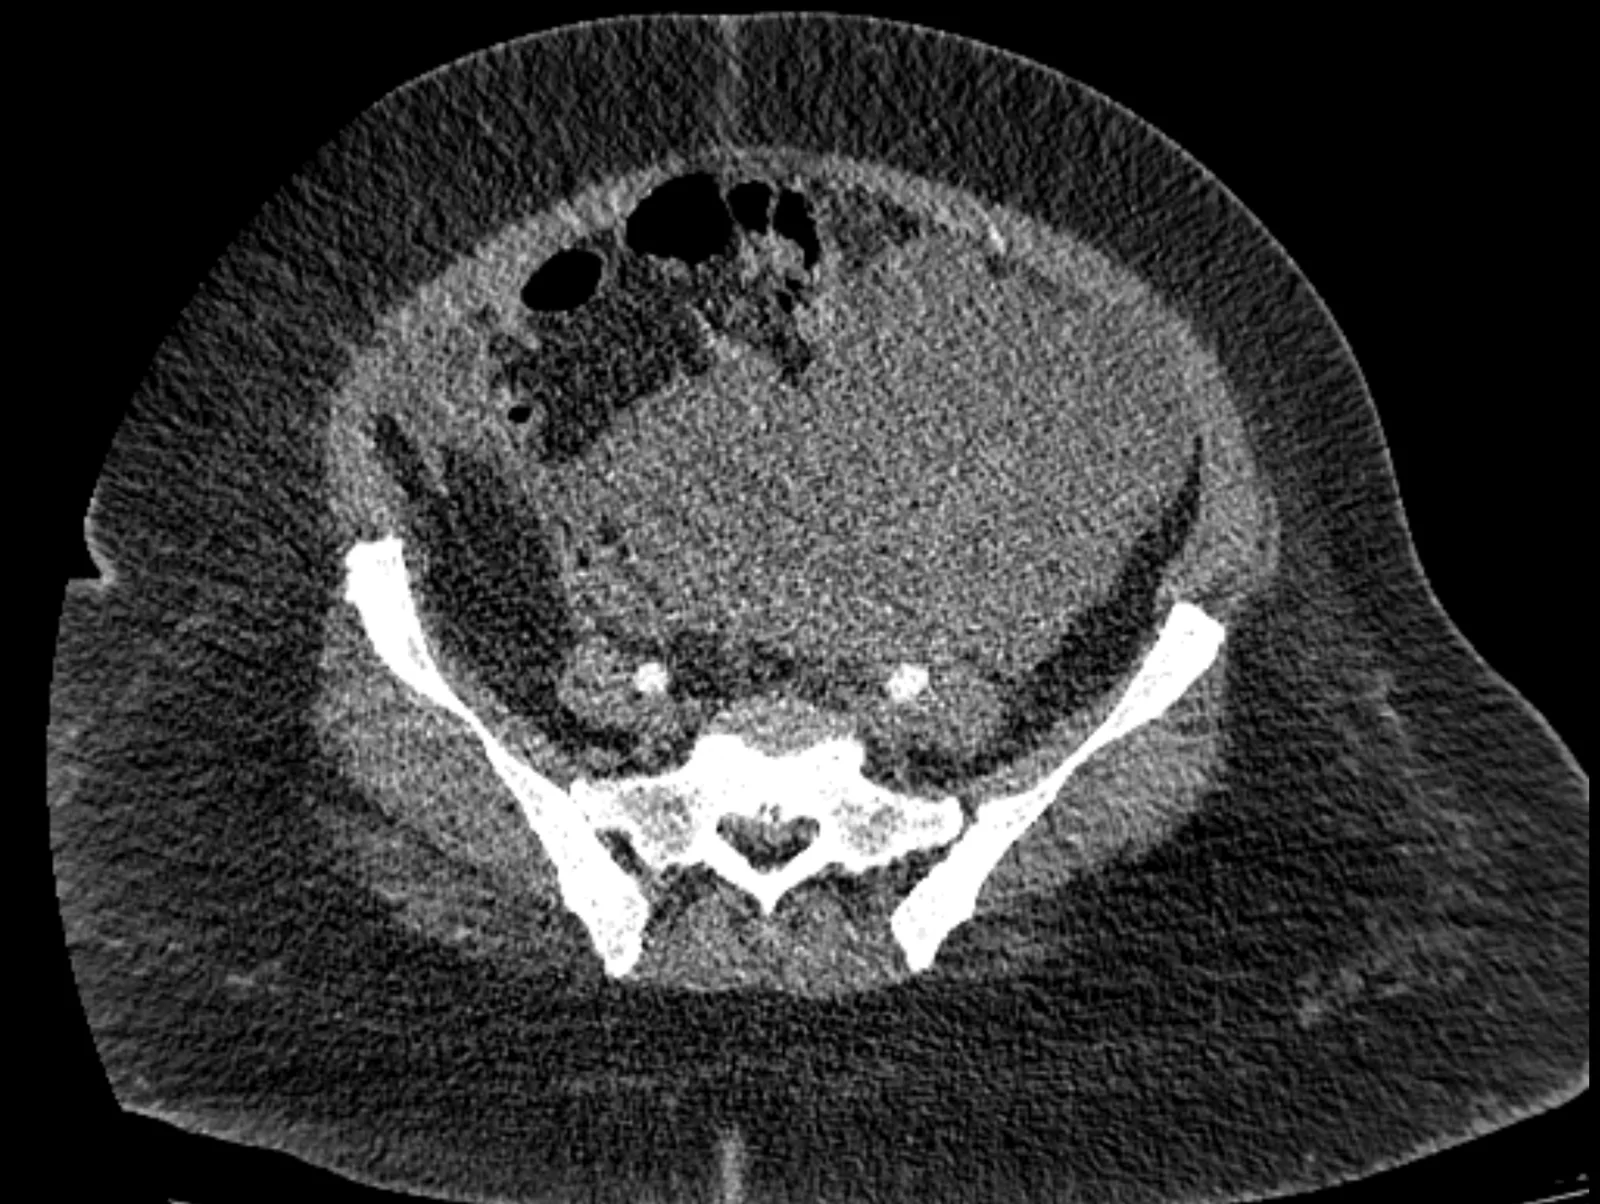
Contrast-enhanced CT of the abdomen and pelvis (coronal view), demonstrating a large heterogeneous pelvic mass measuring approximately 16.2 × 15 × 15 cm, with massive ascites CT: computed tomography
